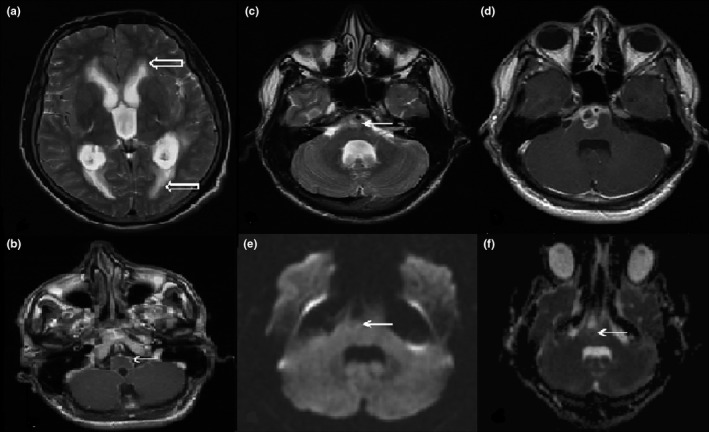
In patient with meningoencephalitis, first MRI axial T2‐weighted and postcontrast axial T1‐weighted images demonstrated (a) a tetraventricular hydrocephalus, transependymal CSF transition, and (b) leptomeningeal enhancement especially in the basal sections and brainstem levels. Follow‐up (12th day) T2‐weighted MRI revealed heterogeneous hyperintense signal changes in the pons and right hemisphere (c). In the repeated MRI after worsening of the patient's clinical findings, (24th day) there occurred a heterogenous area with lobulated peripheral contrast enhancement in the T1‐weighted scans (d) with minimal hyperintensity and restriction in the diffusion and ADC map sequences (e–f) in the pons
